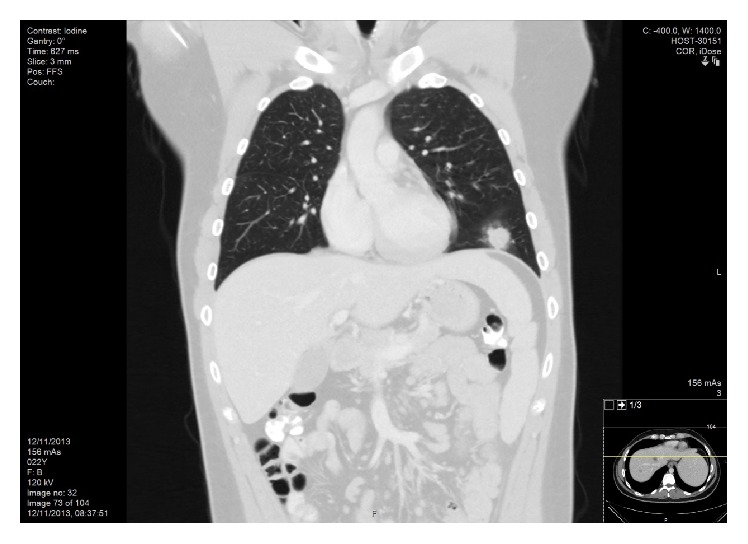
Coronal reconstruction of thoracic CT scan in lung window irregular pulmonary nodule with surrounding ground glass opacity in the left lower lobe of the lung.
